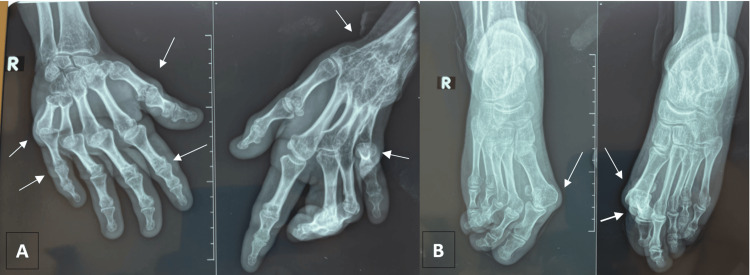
X-ray of the hands and feet. A. Hands X-ray showing flattening and epi-metaphyseal enlargement of the metacarpophalangeal and proximal interphalangeal joints and ankylosis of the left radiocarpal, intercarpal, and carpometacarpal joints. B. Feet X-ray revealing bilateral subluxation of the first metatarsophalangeal joint, along with subluxation of the second and third metatarsophalangeal joints on the right foot, shortening of the left first intermediate phalanx, and brachymetatarsia of the fourth metatarsal.
